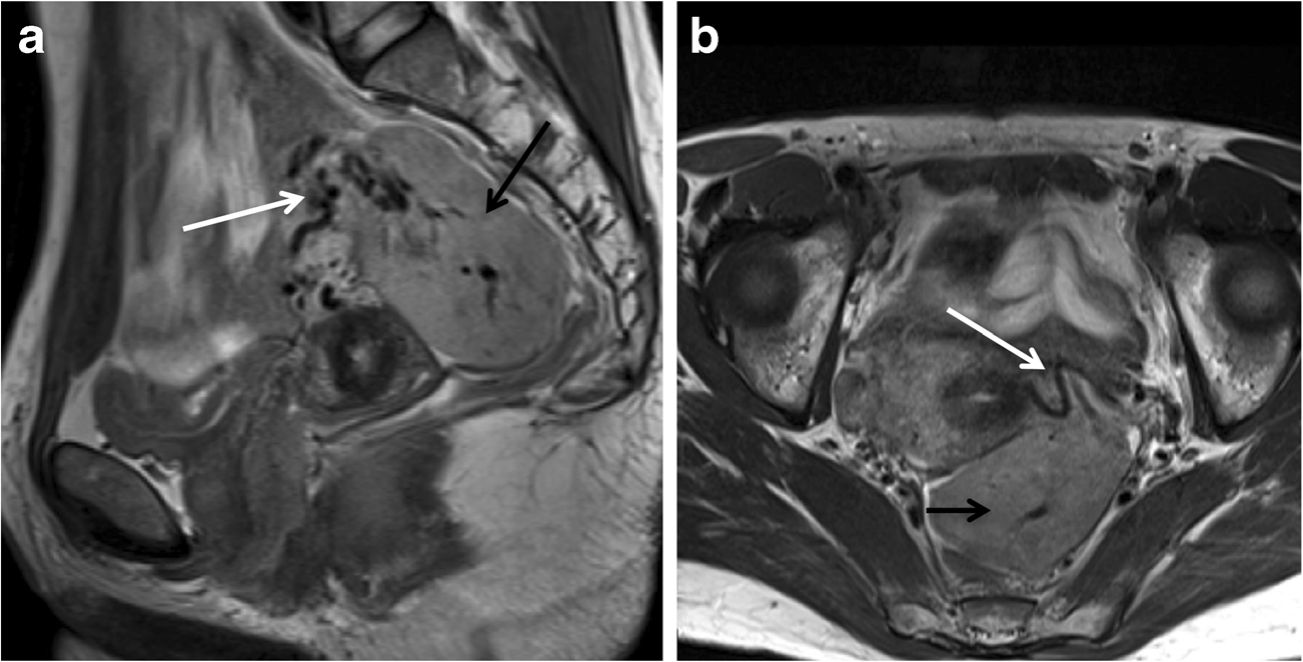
Sagittal and axial T2-weighted images showing a pedunculated subserosal leiomyoma (MRI FIGO 7) within the pouch of Douglas (black arrow). The bridging vessels sign confirms the uterine origin of the mass (white arrow)
